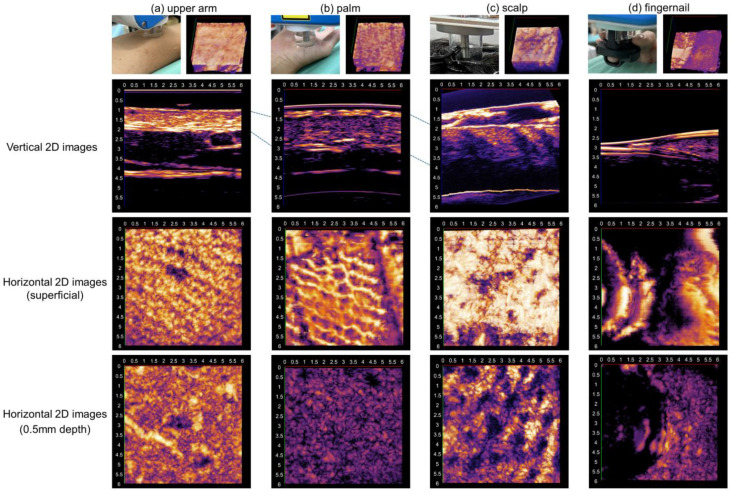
3D-HFUS ultrasonography of healthy skin of different anatomical sites: scanning scenes, 3D original images, and vertical and horizontal 2D images (superficial and 0.5 mm depth) were shown for (a) upper arm, (b) palm, (c) scalp, and (d) fingernail.
